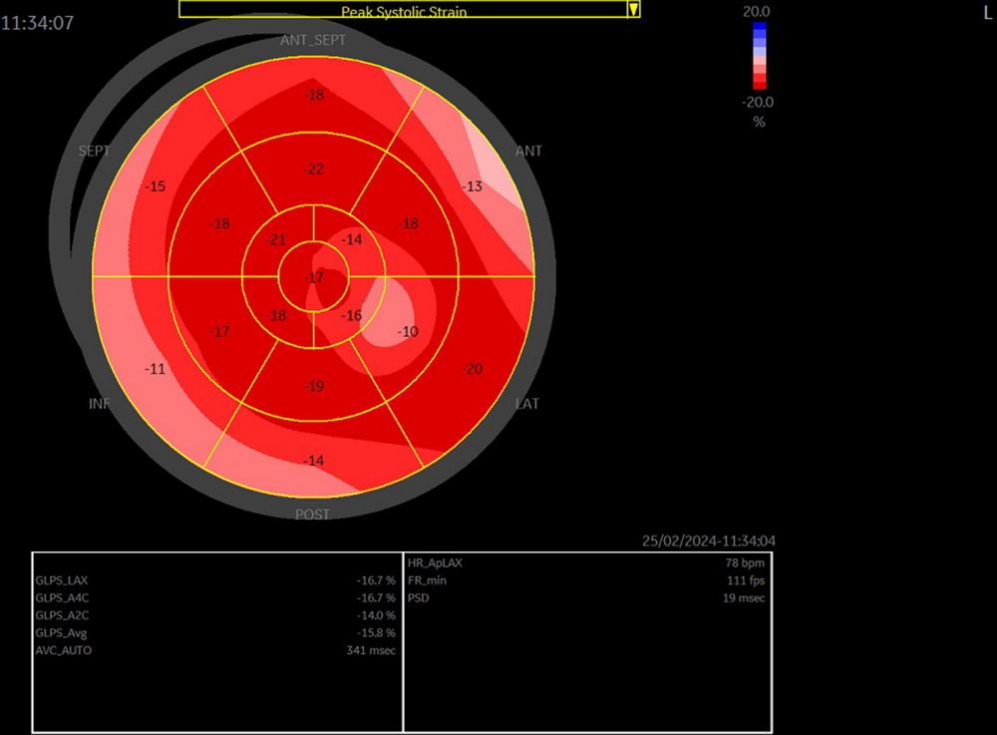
Strain echocardiography (‘bulls eye’)—the mildly decreased global longitudinal strain in the inferior wall, anterior wall, and apex.
